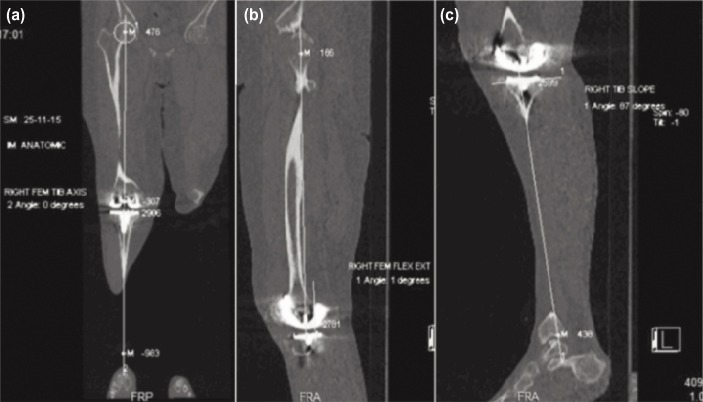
Post-operative measurements of (a) Hip-Knee Angle, (b) Sagittal Femoral Angle and (c) Sagittal Tibial Angle on CT radiographs using the CT Perth Protocol.
